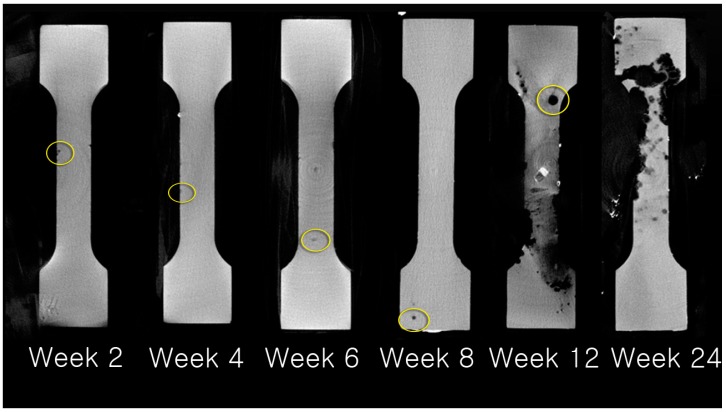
Pattern of resorption of HA-Mg plates examined using micro-computed tomography. Resorption had pin-point-like appearance until week 8 (yellow circles). Resorption progressed rapidly beginning at week 12.
